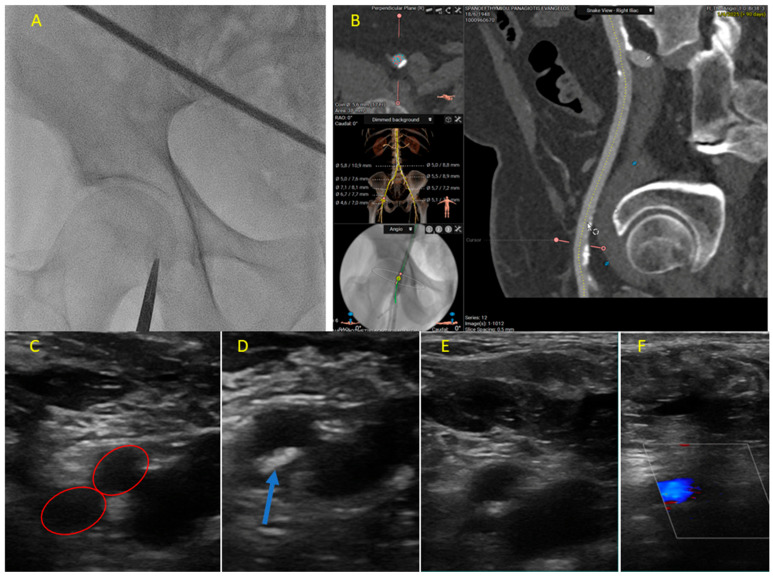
Multimodality imaging for femoral access puncture in patients undergoing transcatheter aortic valve implantation. (A) Detection of the head of the femoral bone via fluoroscopy. Forceps can be used to easily indicate the middle segment of the femoral head as the ideal point for puncture at the middle third of the common femoral artery. (B) Co-registration of the fluoroscopy image with the CT angiography. A calcified atherosclerotic segment of the common femoral artery was detected by CT angiography (red lines) and projected over the fluoroscopy image (yellow circle). In addition, the site of the bifurcation between the profunda and the superficial femoral artery is noted below the middle third of the femoral head. Finally, we can clearly identify the optimal site for arterial puncture just at the beginning of the atherosclerotic plaque, corresponding to just above the mid-level of the femoral head. (C) The point of bifurcation into the superficial femoral artery and profunda (red circles) was also identified by ultrasound. (D) The area of calcification and atherosclerotic plaque was recognized (blue arrow), and the probe was moved higher based on the initial mark made by the forceps to identify the middle third of the femoral head. (E) The ideal point for puncture above the area of calcified atherosclerotic plaque and at the healthiest segment of the vessel before transition to the external iliac artery was selected. (F) The presence of brisk flow in the common femoral artery was confirmed by color Doppler after final closure (white rectangle).
